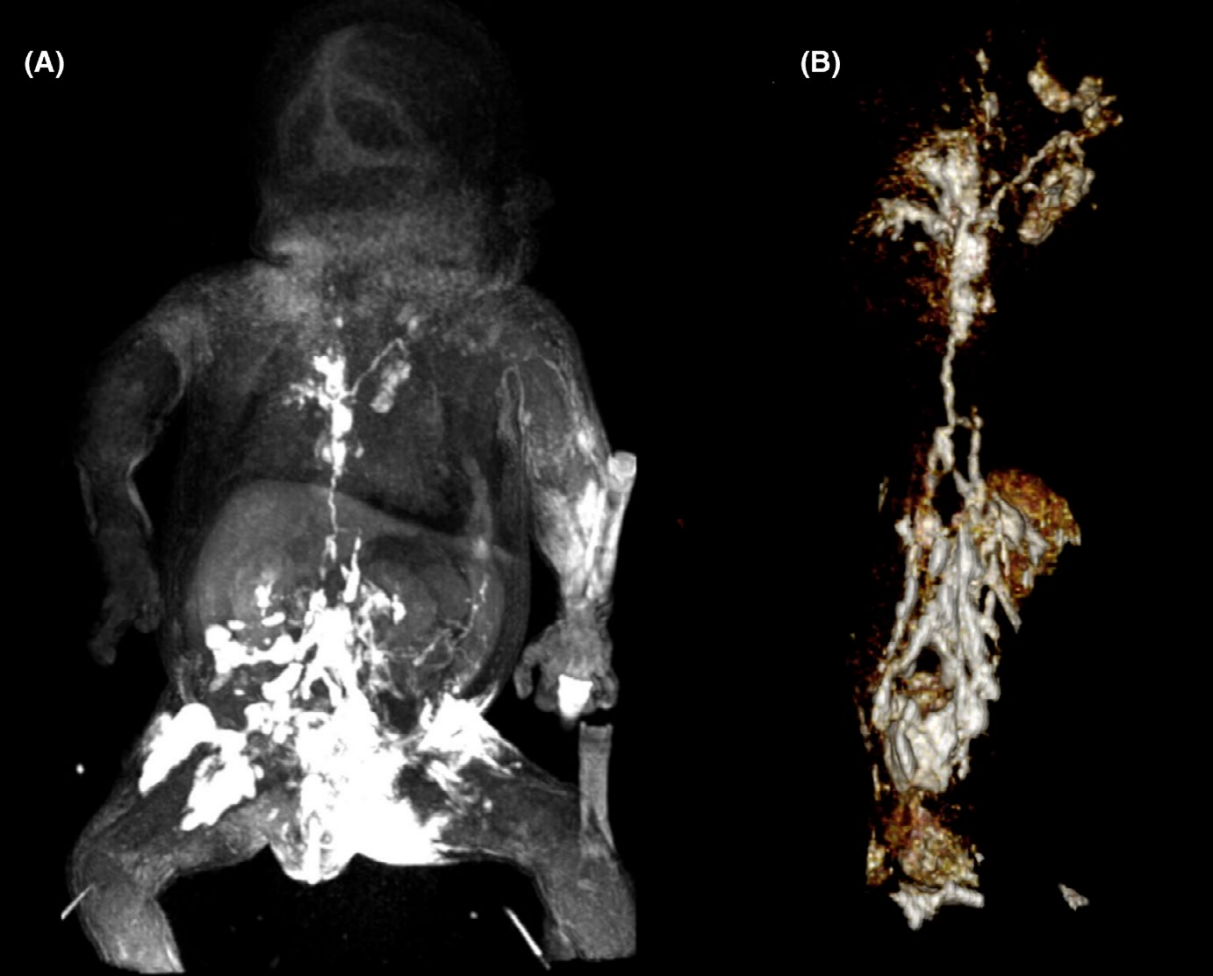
(A) Intranodal dynamic contrast‐enhanced MR lymphangiogram of the abdomen and thorax demonstrating the cisterna chyli lying left of the midline. The thoracic duct is continuous with the cisterna and lies initially to the left of the midline and then immediately crosses to the right aspect of the spine ascending along the right of the spine (with several cystic dilatations) and crossing midline at T5 to its terminus at the left subclavian/innominate vein confluence. (B) MRI 3D reconstruction of lymphatics vessels
